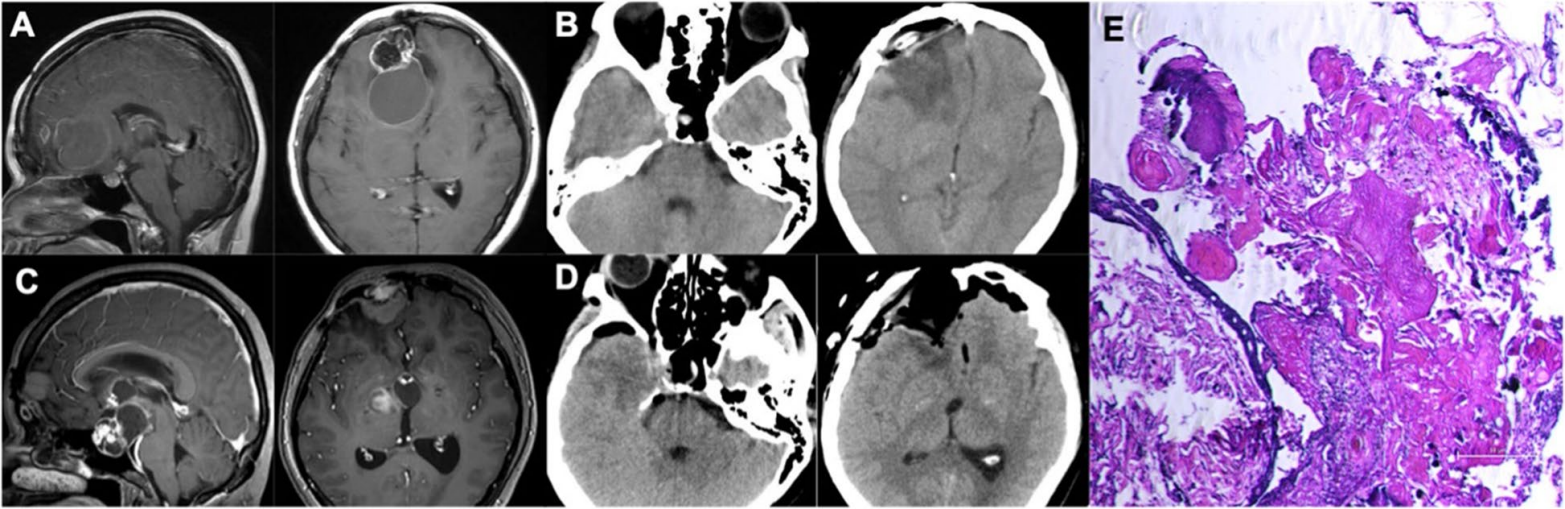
MRI, CT scans, and pathological features of case 1. A Preoperative MRI showed a cystic solid lesion in the sellar-suprasellar region extending to the right frontal lobe in 2012. B Postoperative CT scan showed total resection of the right frontal lesion. C MRI scan showed tumor recurrence in both the sellar-suprasellar region and the right frontal lobe in 2021. D Postoperative CT scan showed total resection of the two lesions. E The pathological features showed typical morphology of adamantinomatous craniopharyngioma
